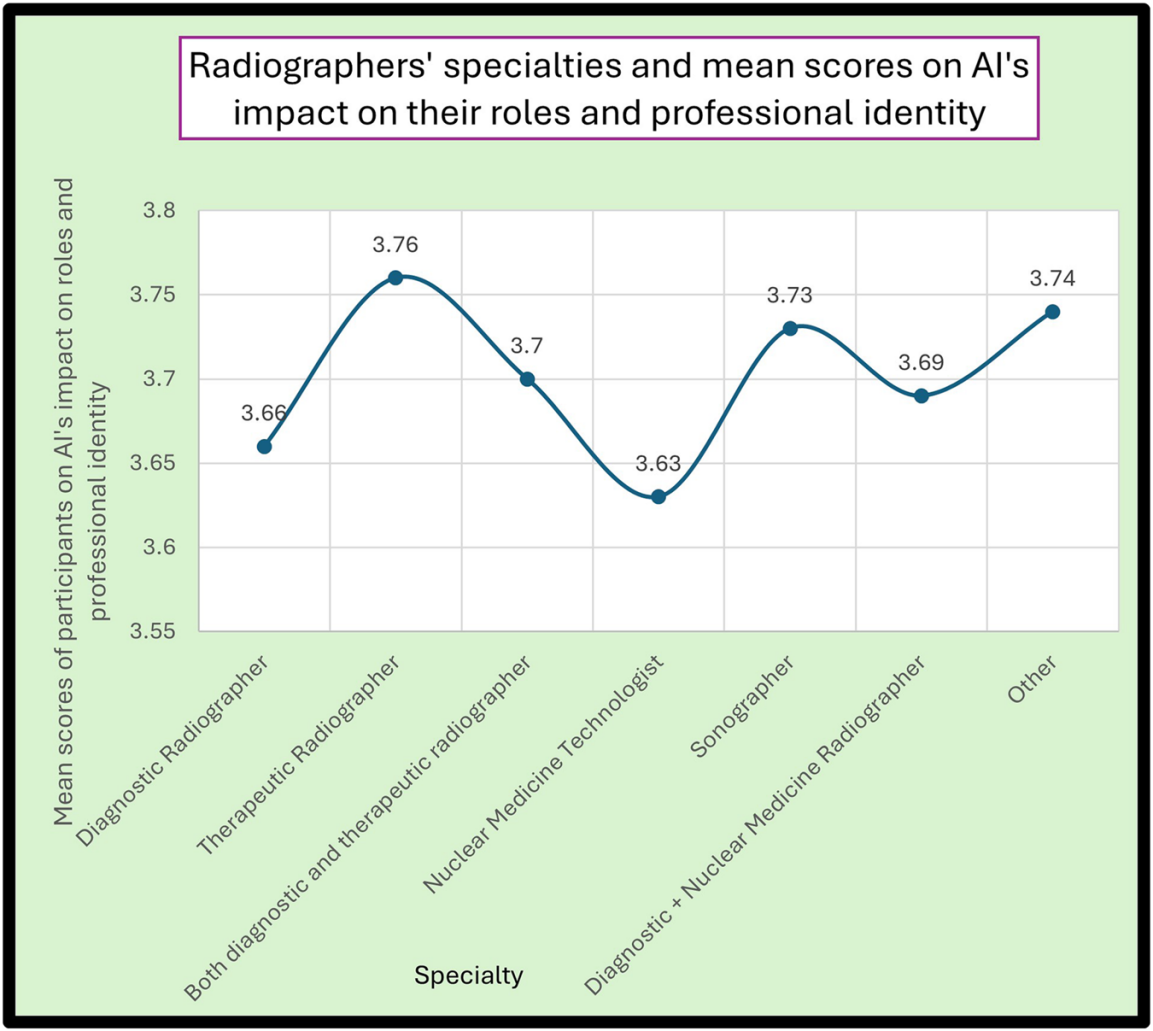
Radiographers’ specialties and mean scores on AI’s impact on their roles and professional identity
